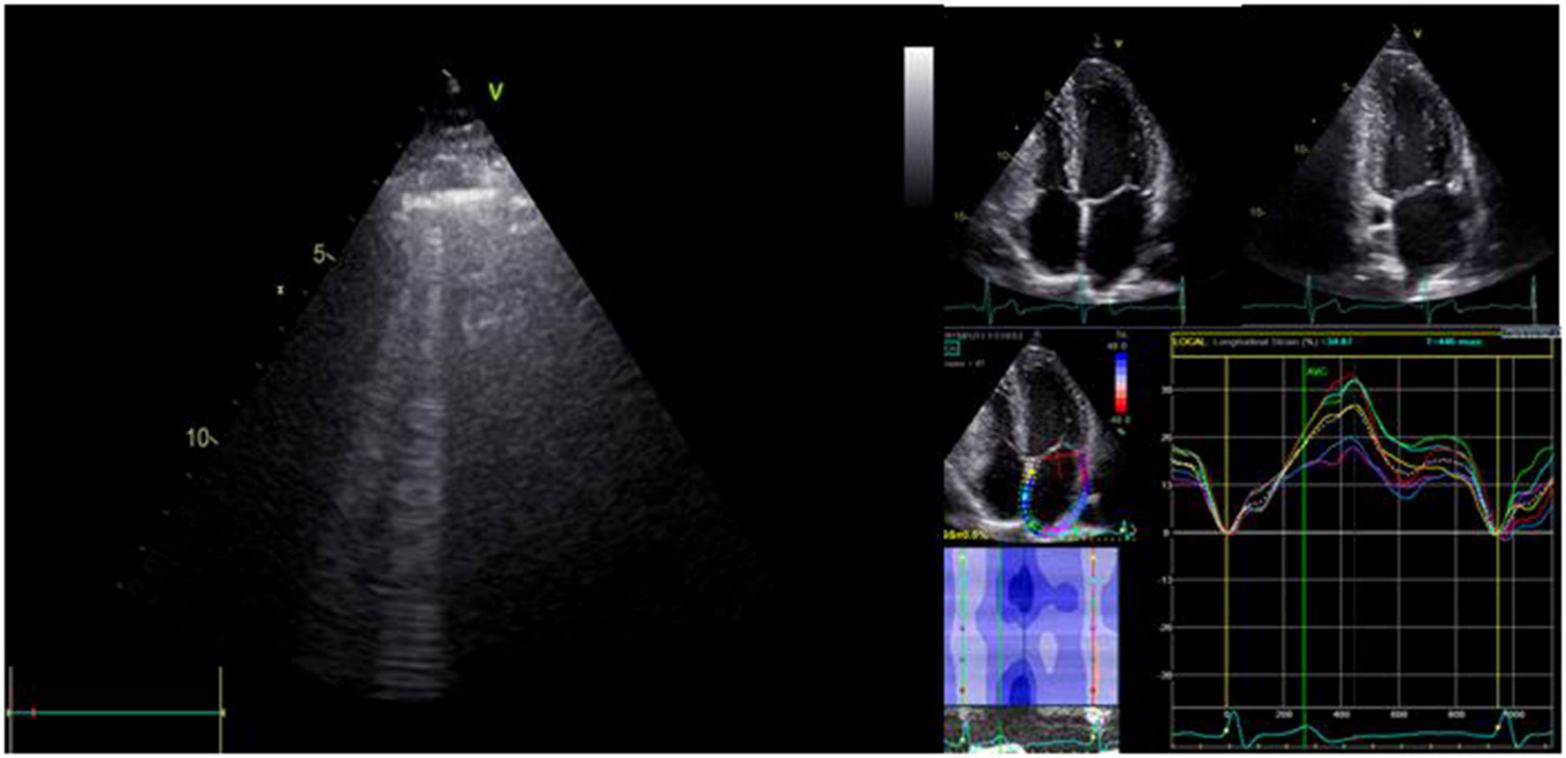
Assessment of B-lines by lung ultrasound and determination of left atrial reservoir strain (LASr) in patients with HFpEF.
